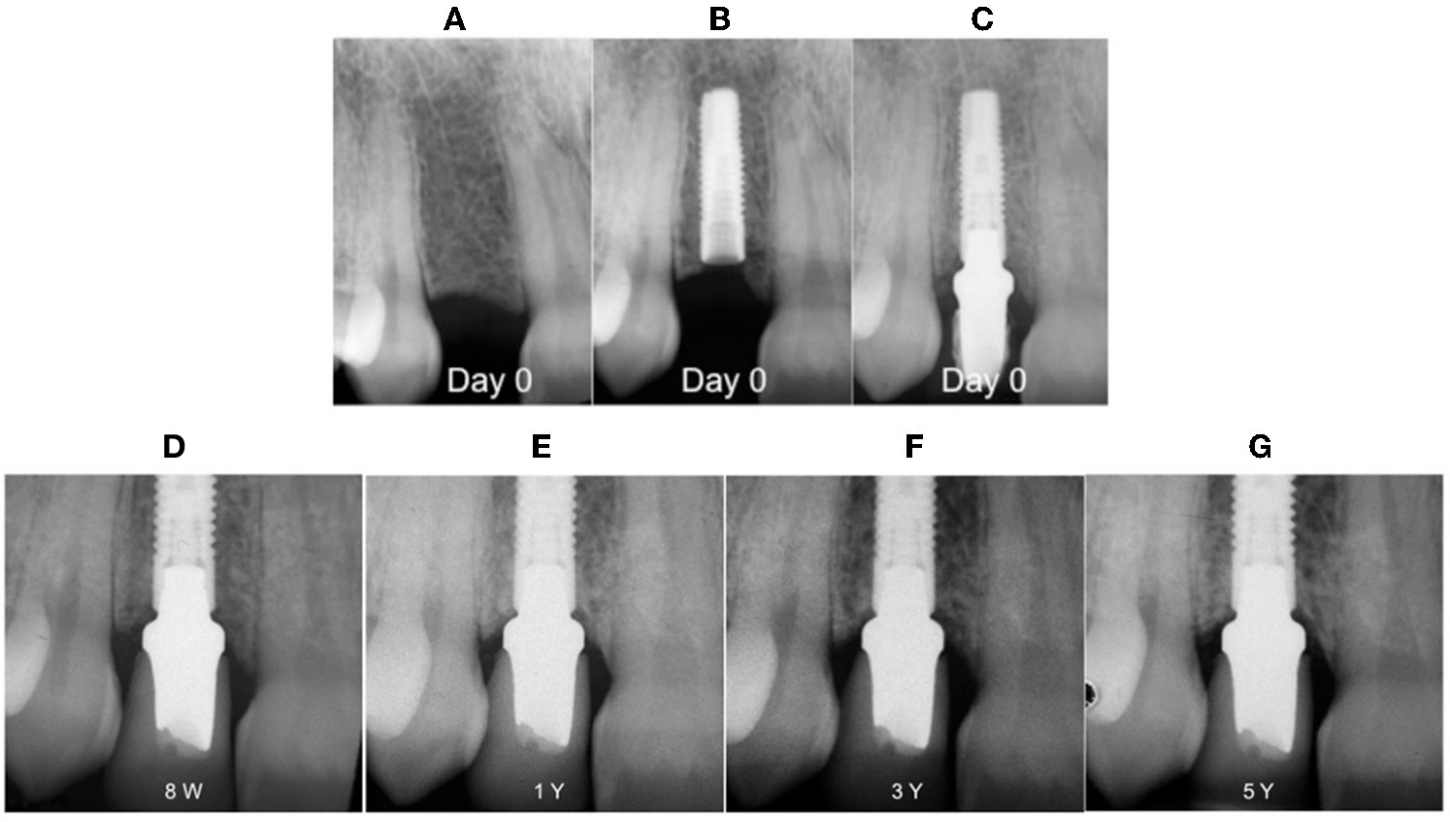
Digital intra-oral radiographs of implants placed in healed ridge. (A) Pre-operative situation; (B) implant placement; (C) abutment placement; (D) 8 weeks after definitive crown cementation; (E) after 1 year; (F) after 3 years; (G) after 5 years.
